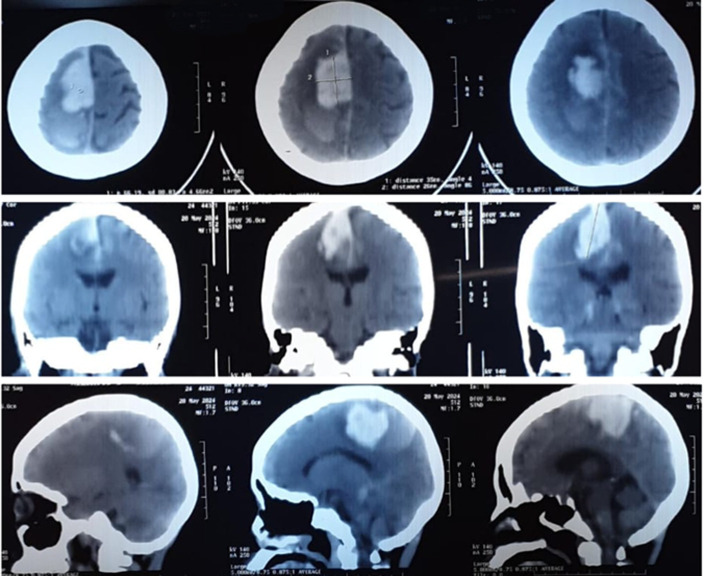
computed tomography of the head without contrast demonstrating a large intraparenchymal hemorrhage centered in the right parietal lobe area
